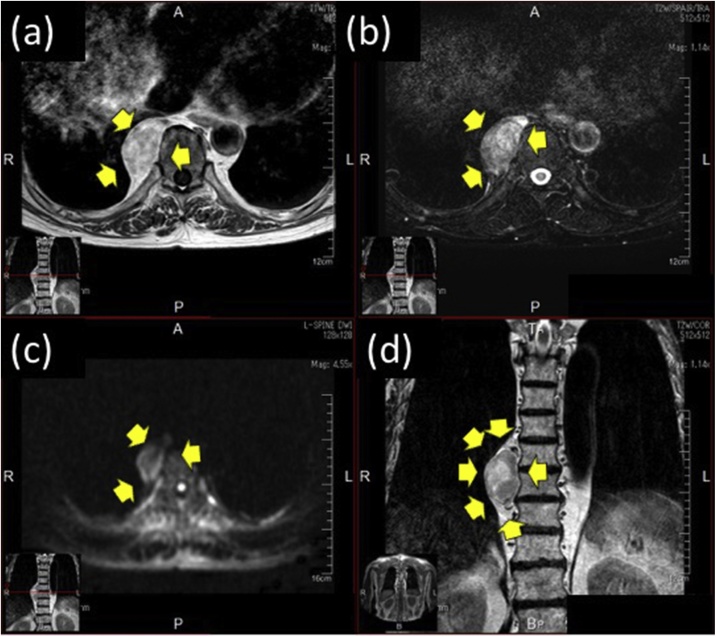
Magnetic resonance images of the posterior mediastinal tumor. (A) The T1 weighted image. (B) The T2 weighted image. (C) Diffusion-weighed image. (D) Magnetic resonance image (coronal plane) reveals the mass attached to the T9 thoracic vertebra.
